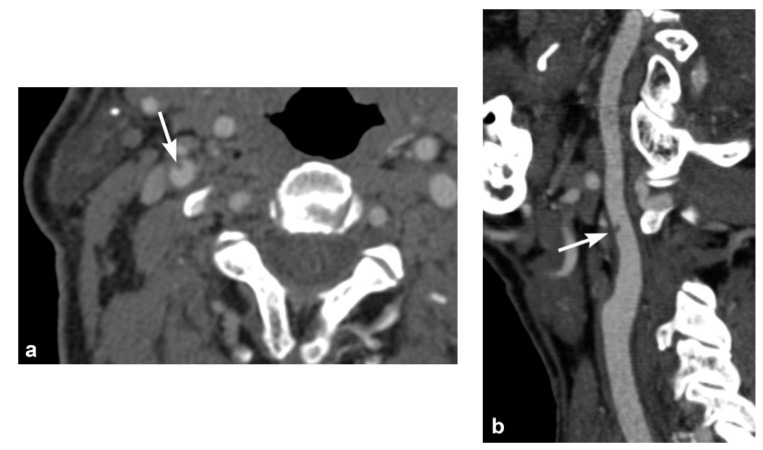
Right internal carotid artery (ICA) injury in a 73-year-old male involved in a motor vehicle accident. (a) Axial and (b) sagittal oblique multiplanar reformatted multidetector CT angiographic images show a small raised intimal flap in the proximal right ICA.
